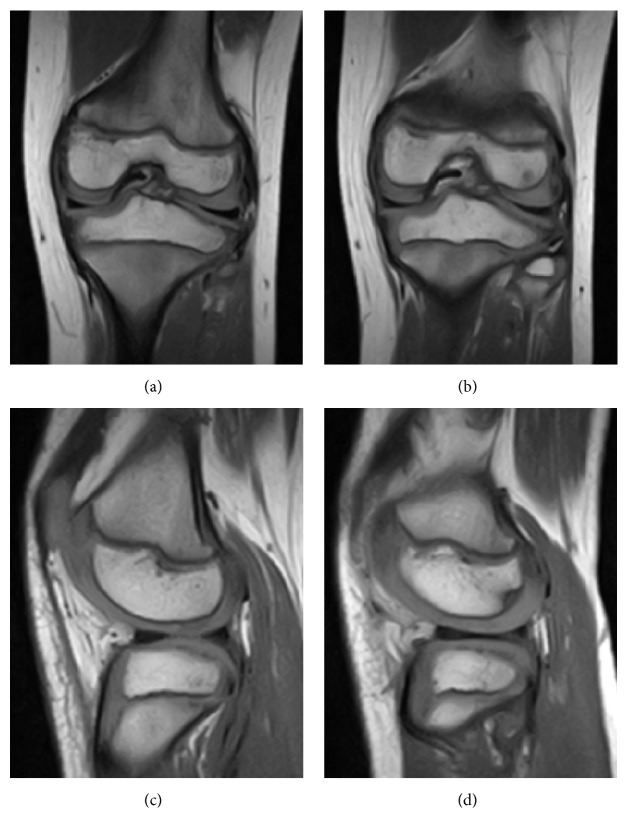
T1-weighted magnetic resonance images. Coronal (a, b) and sagittal (c, d) sections showing normal findings of the lateral meniscus.
